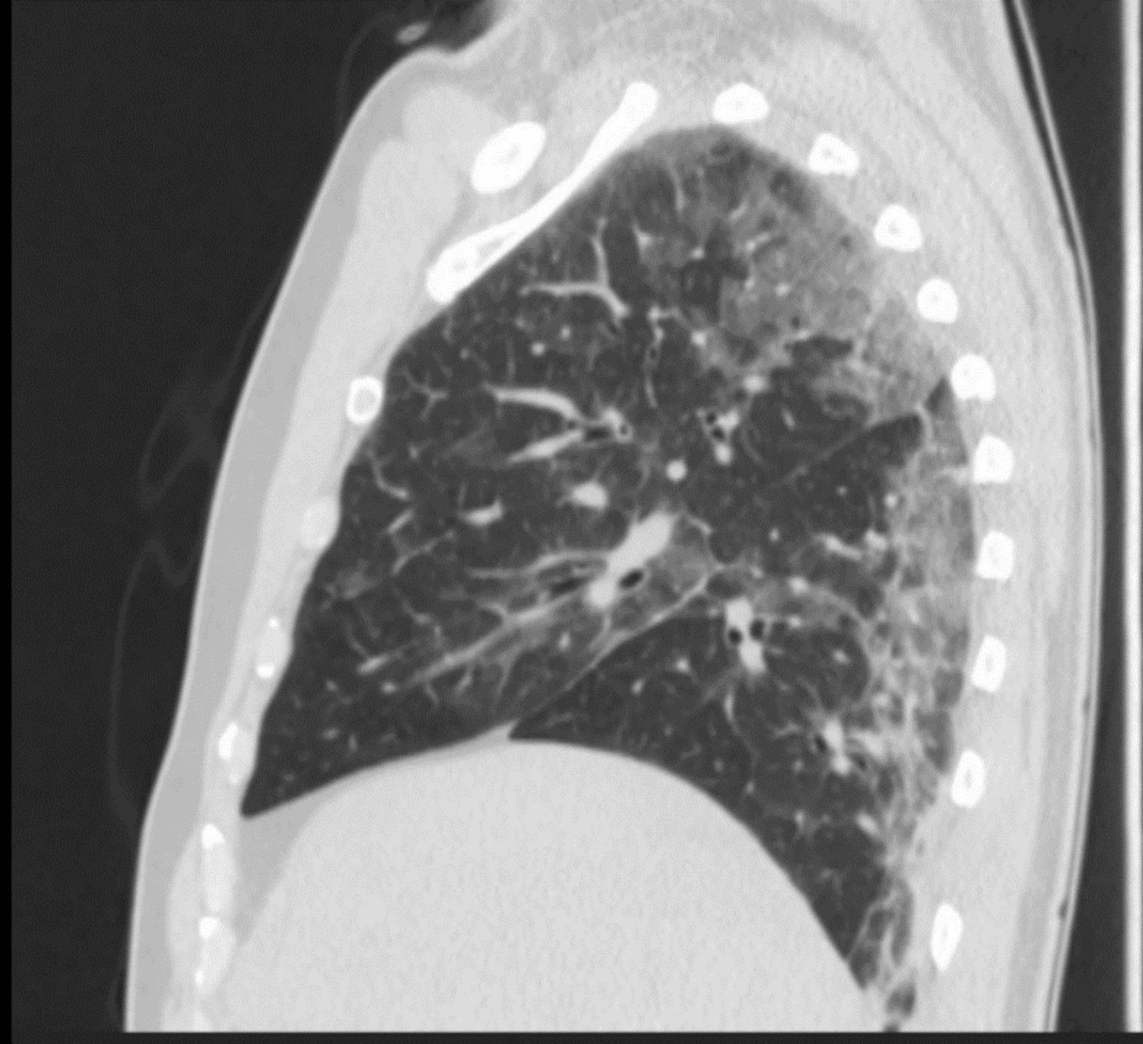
Gravity-dependent lung involvement in the chest CT
